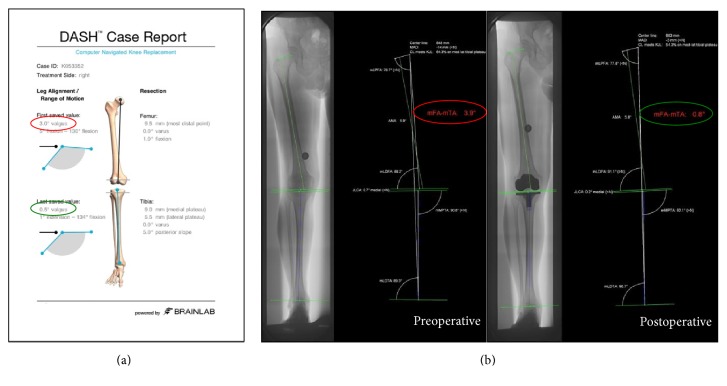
(a) Patient report generated by the navigation system after surgery. (b) Pre- and postoperative full-length weight-bearing radiographs. Axial limb alignment was measured using the planning tool mediCAD.
